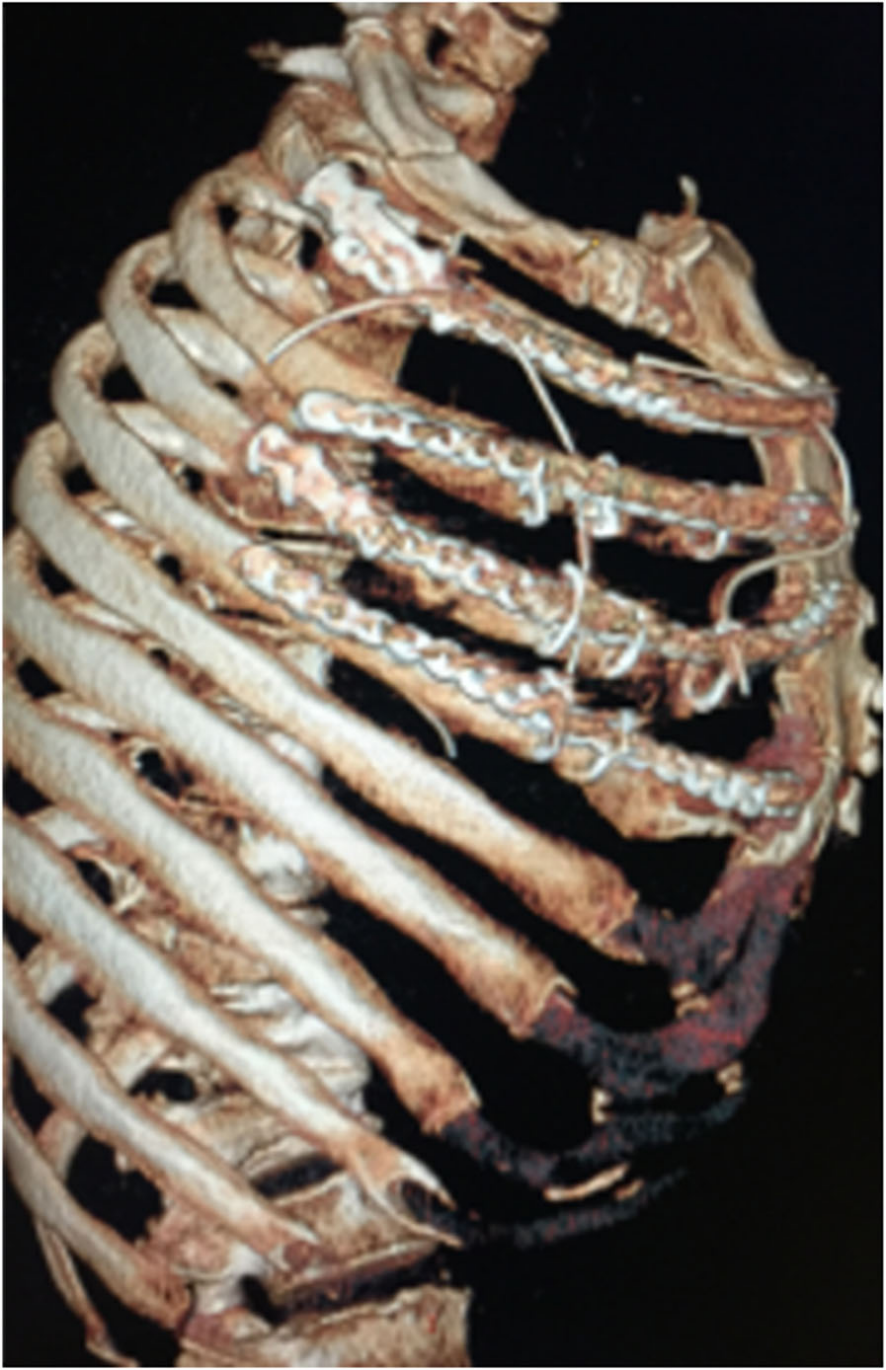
The locking plate reviewed by rib CT after surgery was consistent with that in preoperative plan
